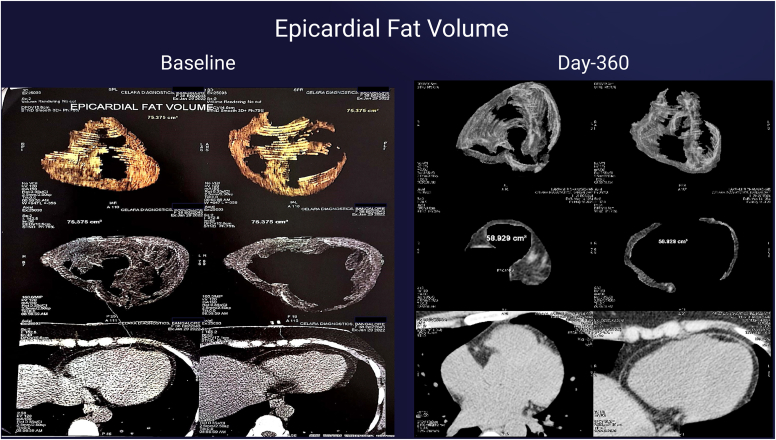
Epicardial fat volume measured by computed tomography (CT) scan at baseline and day 360 epicardial volume decreased from 75.3 cm3 at baseline to 58.92 cm3 at day-360 (21.8% reduction).
